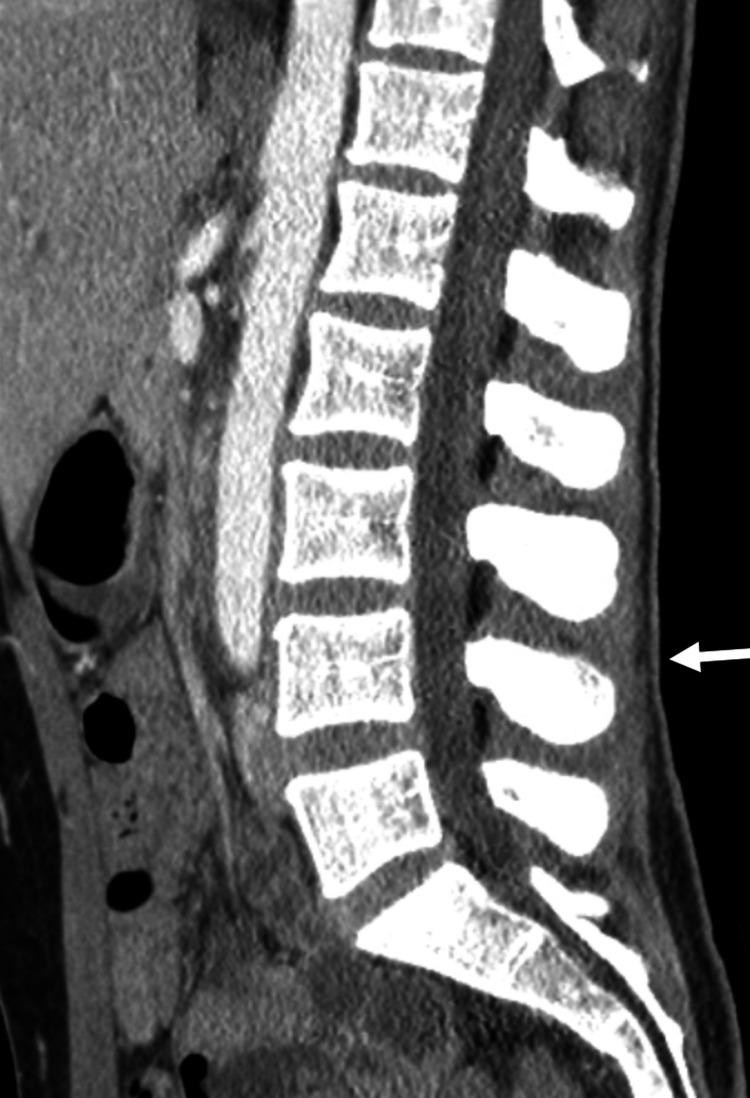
Sagittal lumbar spine computed tomography with contrast, showing nonspecific mild induration of the subcutaneous fat overlying the L3/L4 spinous processes (white arrow)
